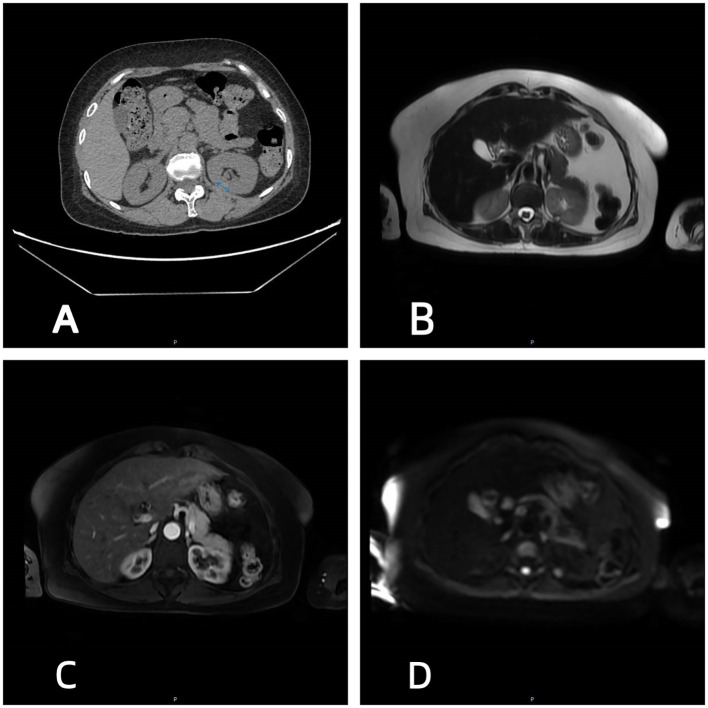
CT and MRI imaging of PRNRP.
